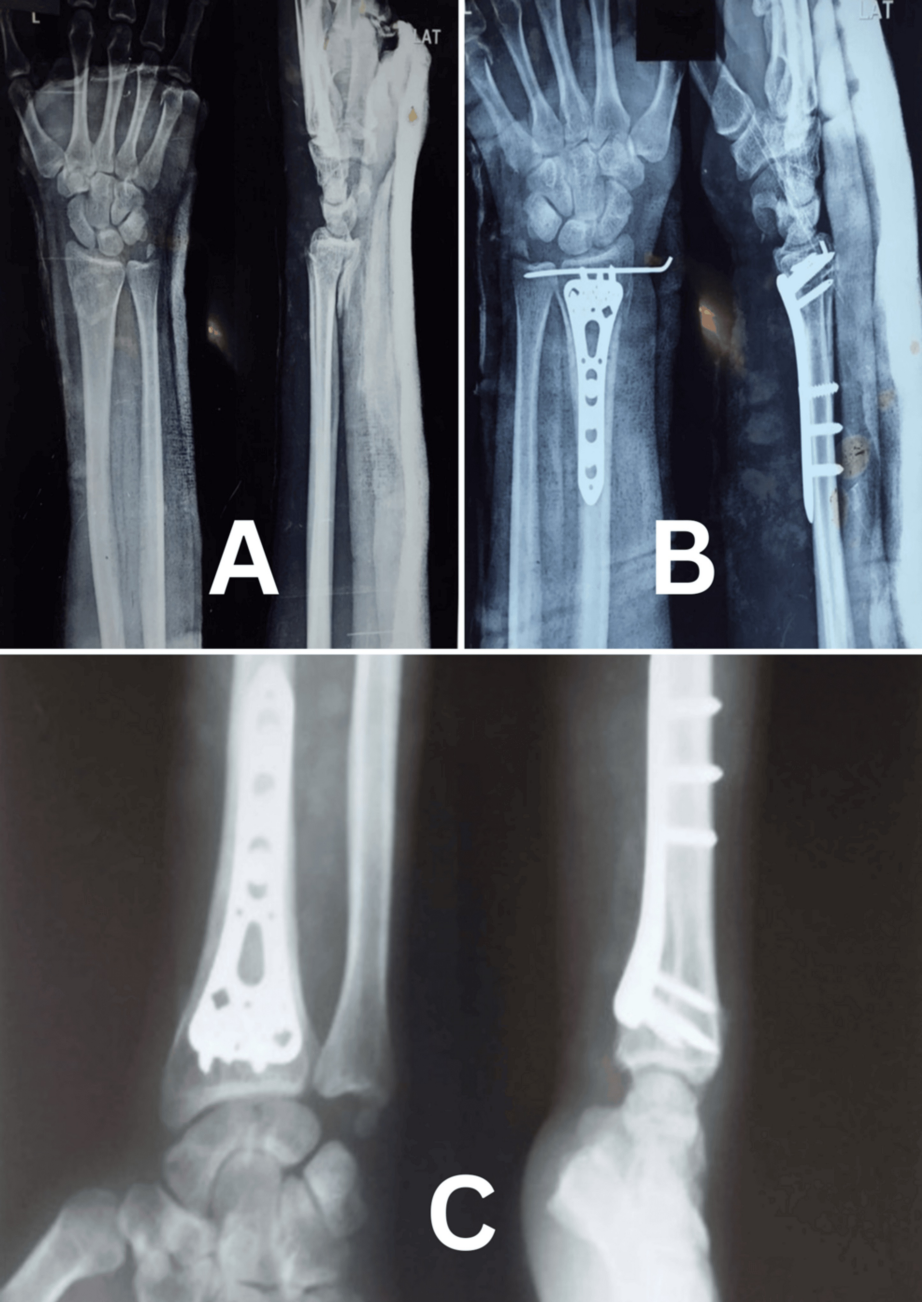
Case 2 wrist X-rays (A) Preoperative anteroposterior and lateral wrist X-rays showing a distal radius fracture. (B) Postoperative anteroposterior and lateral wrist X-rays showing fracture fixation. (C) Six-month follow-up anteroposterior and lateral X-rays showing fracture union.
